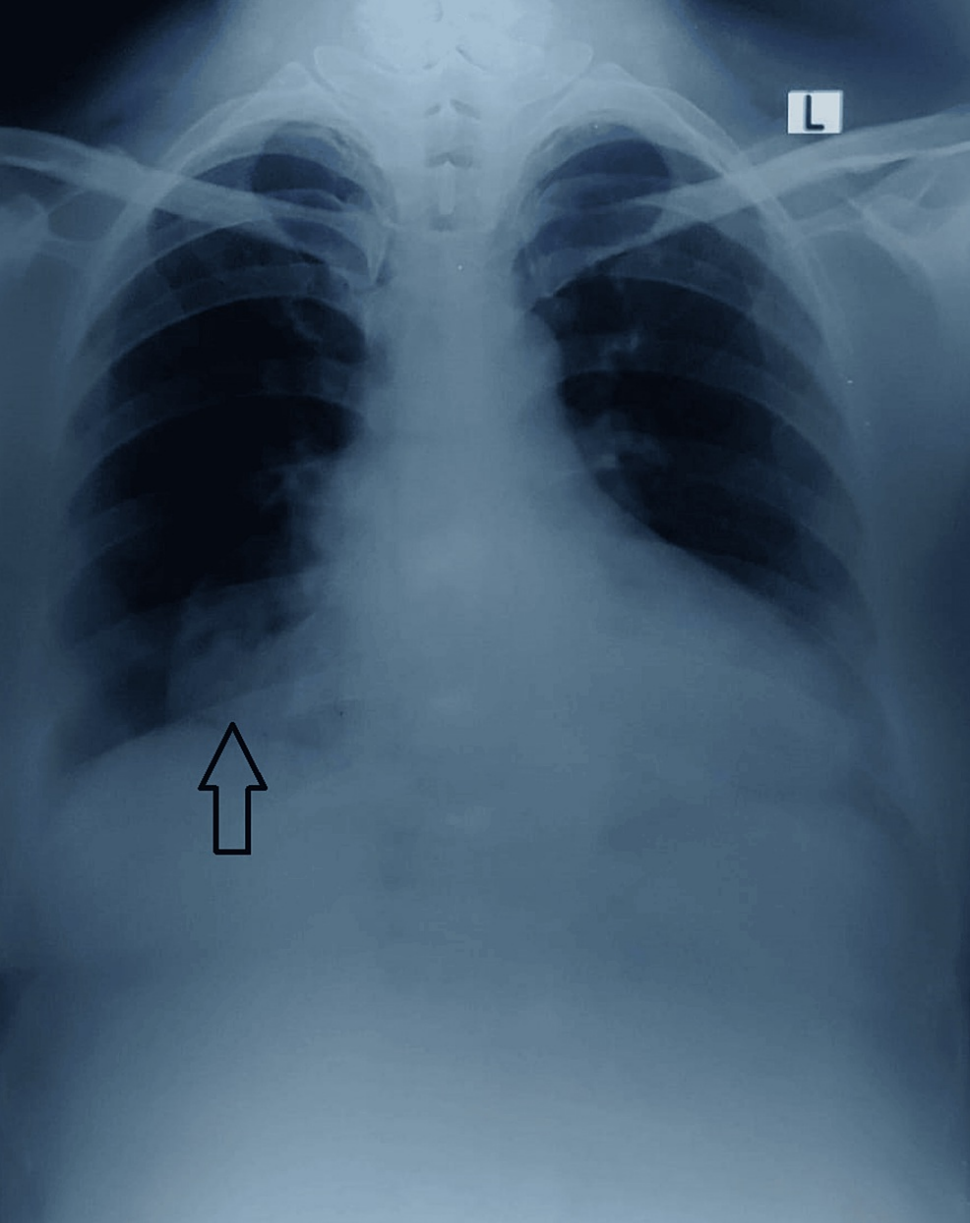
Chest X-ray posteroanterior view - right lower zone, para-cardiac well-circumscribed structure suggestive of a cyst with air-fluid level inside
